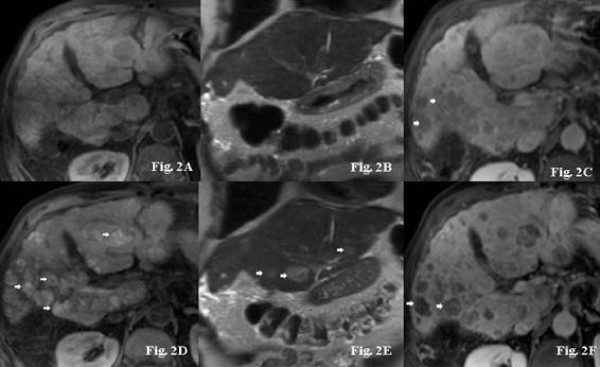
A-F. 71-year-old male patient with multifocal HCC (patient #9). Axial nonenhanced fat-suppressed T1WI imaging of the liver performed at baseline showed multiple mild hypointense HCC lesions (arrow) (Figure 2A). On corresponding coronal HASTE (T2-weighted) images, liver tumors are difficult to distinguish from adjacent liver parenchyma because of signal isointensity (Figure 2B). Fat-suppressed post-Gd SGE imaging showed an almost homogenous signal (arrows) of moderately enhancing liver tumors (Figure 2C). Three weeks after onset of sorafenib therapy, nonenhanced T1WI imaging revealed multiple focal strongly hyperintense lesions (arrows) presumed to represent hemorrhagic necrosis in the known tumors (Figure 2D). Coronal HASTE images performed at the same time demonstrated also hyperintense signals (arrows) of tumors with a good delineation to adjacent liver parenchyma (Figure 2E). Fat-suppressed post-Gd SGE imaging showed central >75% necrosis in most of the HCC lesions following sorafenib therapy (Figure 2F).
